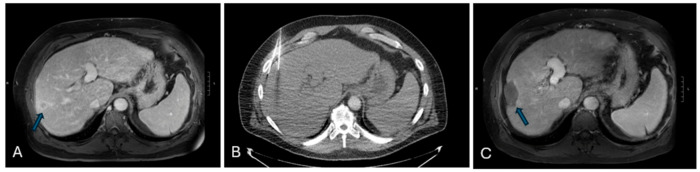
Axial imaging of hepatocellular carcinoma (HCC) treated with microwave ablation (MWA). (A) Pre-procedural contrast-enhanced magnetic resonance imaging (MRI) demonstrates an arterially enhancing lesion with washout (arrow) consistent with HCC in the right hepatic lobe. (B) Intra-procedural computed tomography (CT) showing placement of the microwave ablation probes into the lesion. (C) Post-ablation contrast-enhanced MRI demonstrates a non-enhancing ablation zone (arrow) encompassing the target tumor, consistent with complete treatment.
